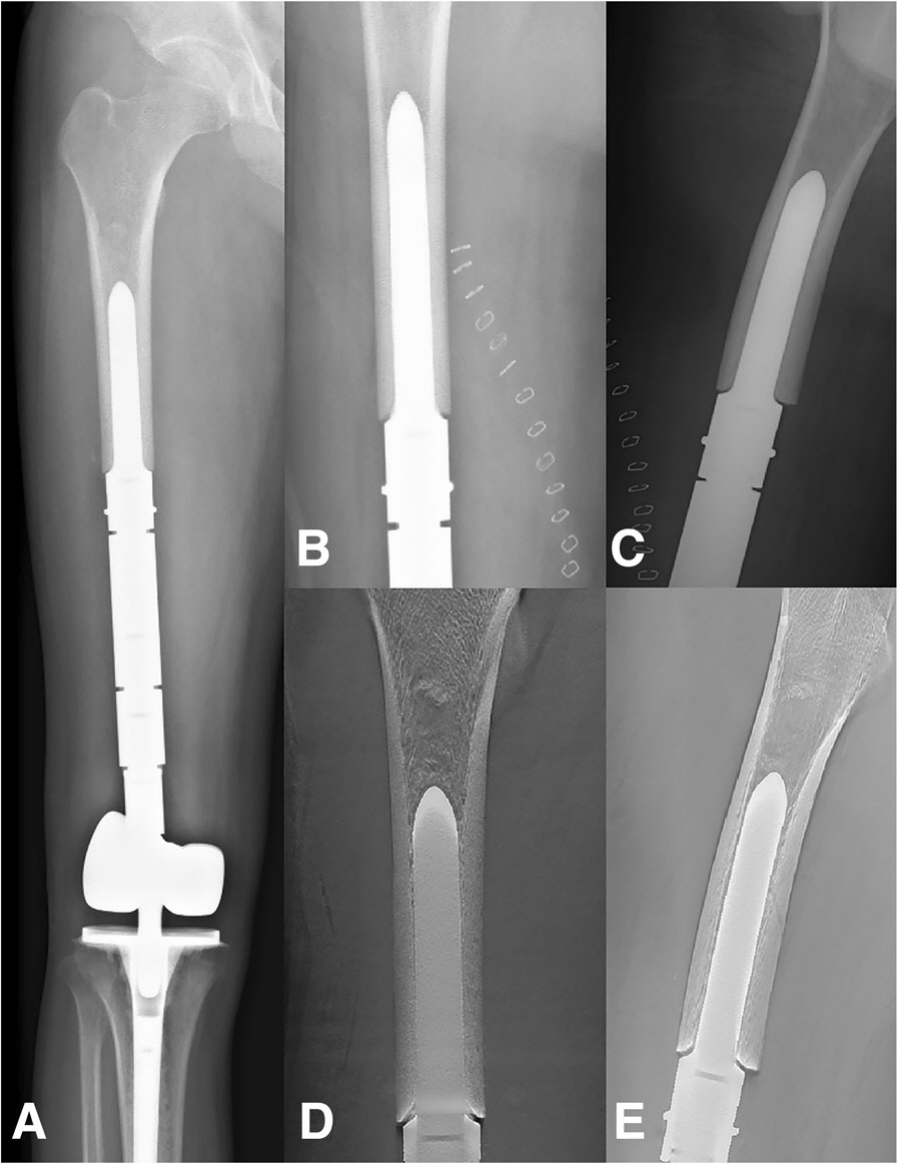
A case of reconstruction of the distal femur following a distal resection of 54% of the length of the femur. a Postero-anterior radiograph of the entire femur. b, c Postero-anterior and lateral radiographic views of the region of stem insertion of the femur. d, e Postero-anterior and lateral T-SMART views of the stem insertion region of the femur
